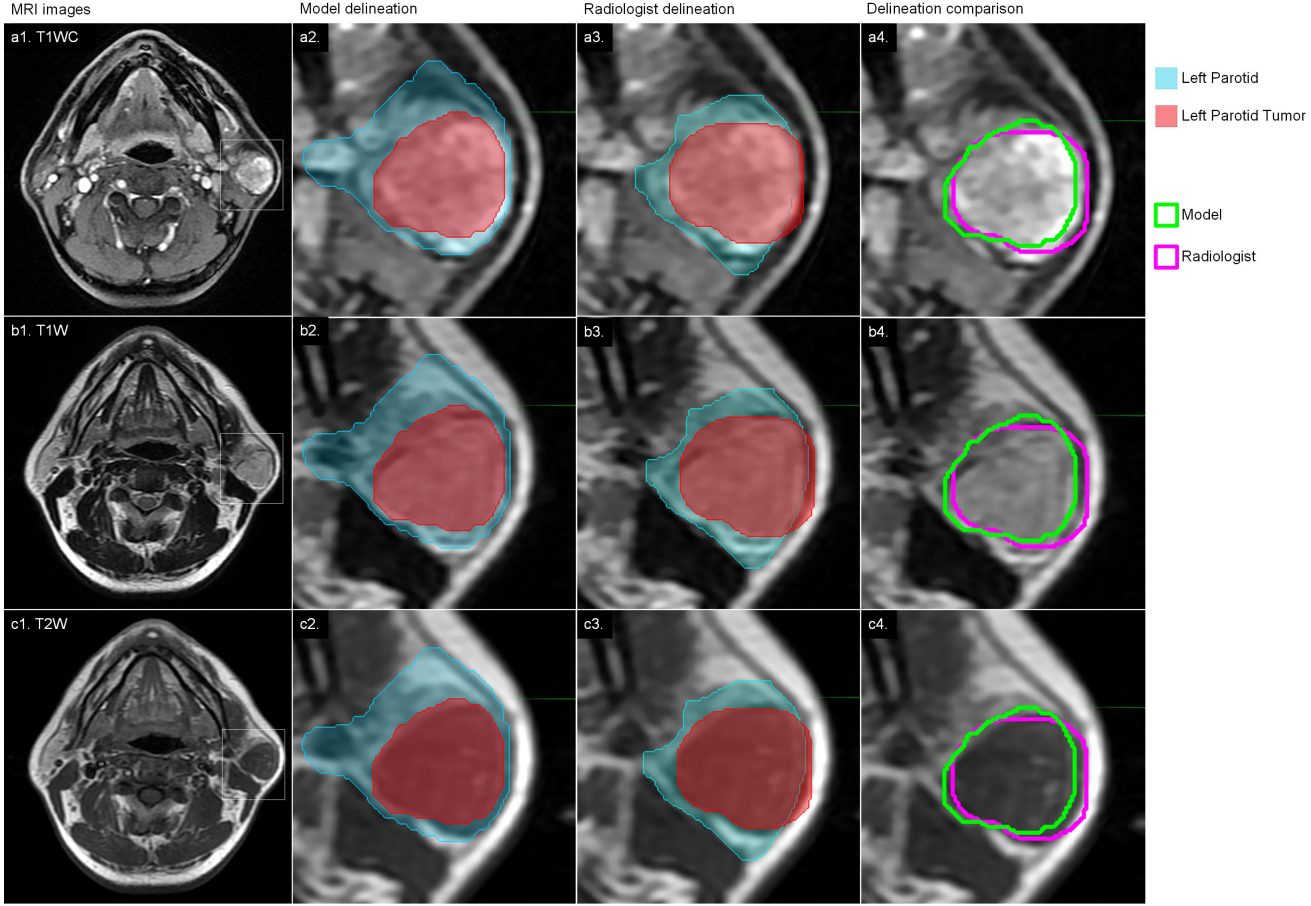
An example of the results. a1, b1 and c1 represent one slice of the MR images; a2, b2 and c2 represent the results of autosegmentation; a3, b3 and c3 represent the results of manual segmentation; a4, b4 and c4 show the comparison of the tumor segmentation.
